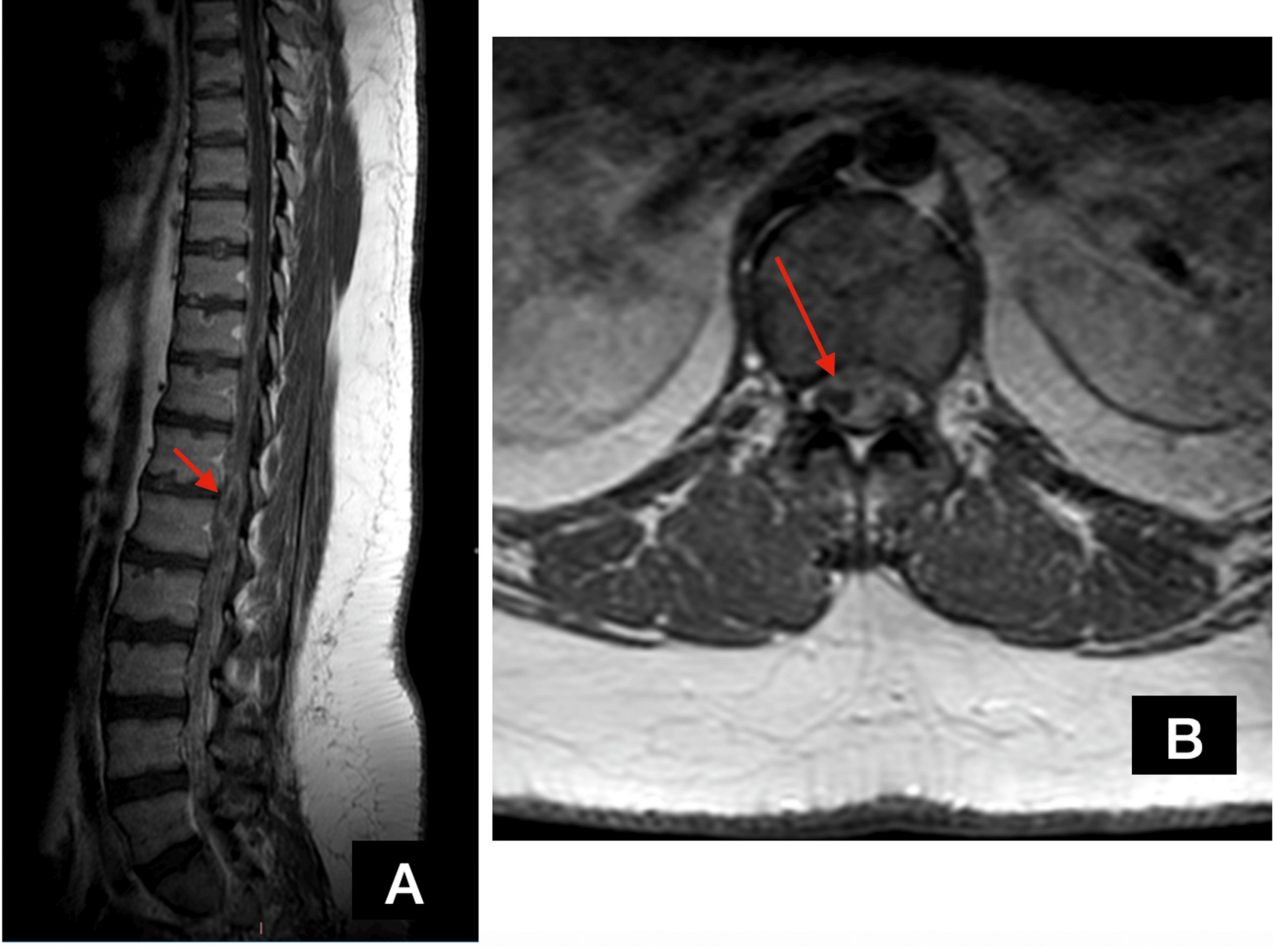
MRI scan findings. A: sagittal post-contrast T1 MRI scan showing a well-defined ring-enhancing lesion at the level of T12-L1; B: axial post-contrast T1 MRI scan of the same lesion
